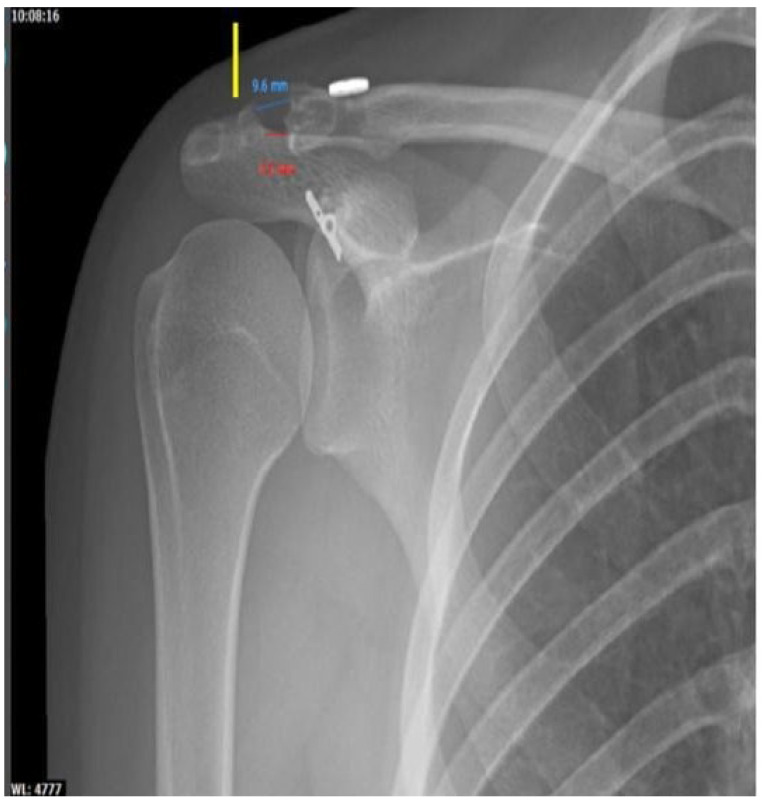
5.5 years post-surgery radiographic control. Correct acromioclavicular alignment, with increased acromioclavicular space. Yellow arrow: vertical bone tunnel of the tendon plasty.
